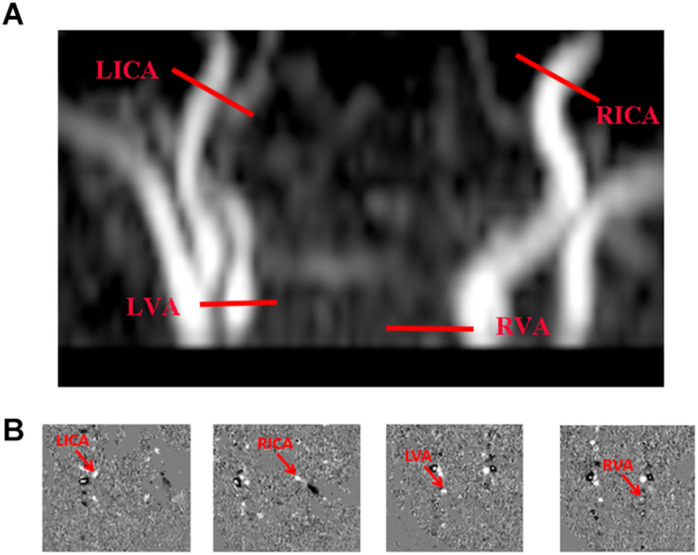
Cerebral blood flow determination via phase-contrast (PC) MRI. (A) Slice positions overlaid on angiogram image (each red bar indicating imaging slice of one PC MRI scan), performing four PC MRI scans in each subject to assess internal carotid arteries (left, LICA; right, RICA) and vertebral arteries (left, LVA; right, RVA), respectively; and (B) Corresponding PC MRI images (delayed).
